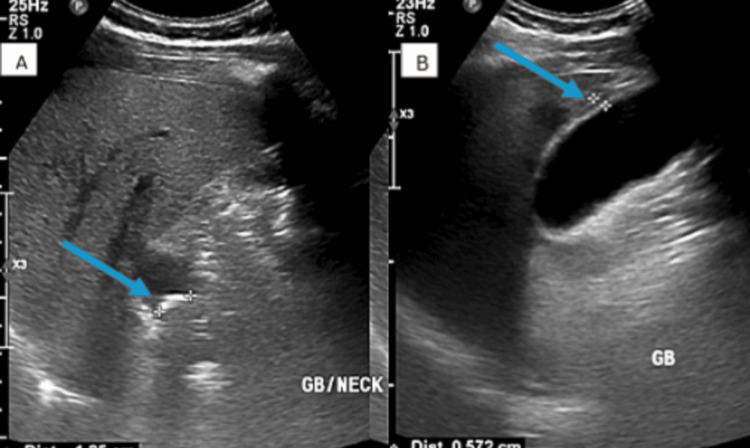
Repeat right upper quadrant ultrasound (longitudinal view). (A) A 1.7 cm obstructing gallstone (arrow) within the gallbladder neck. (B) Thickening of the gallbladder wall measuring 5.7 mm (blue arrow). The liver parenchyma demonstrates diffusely increased echogenicity consistent with hepatic steatosis. No focal hepatic lesions are identified.
